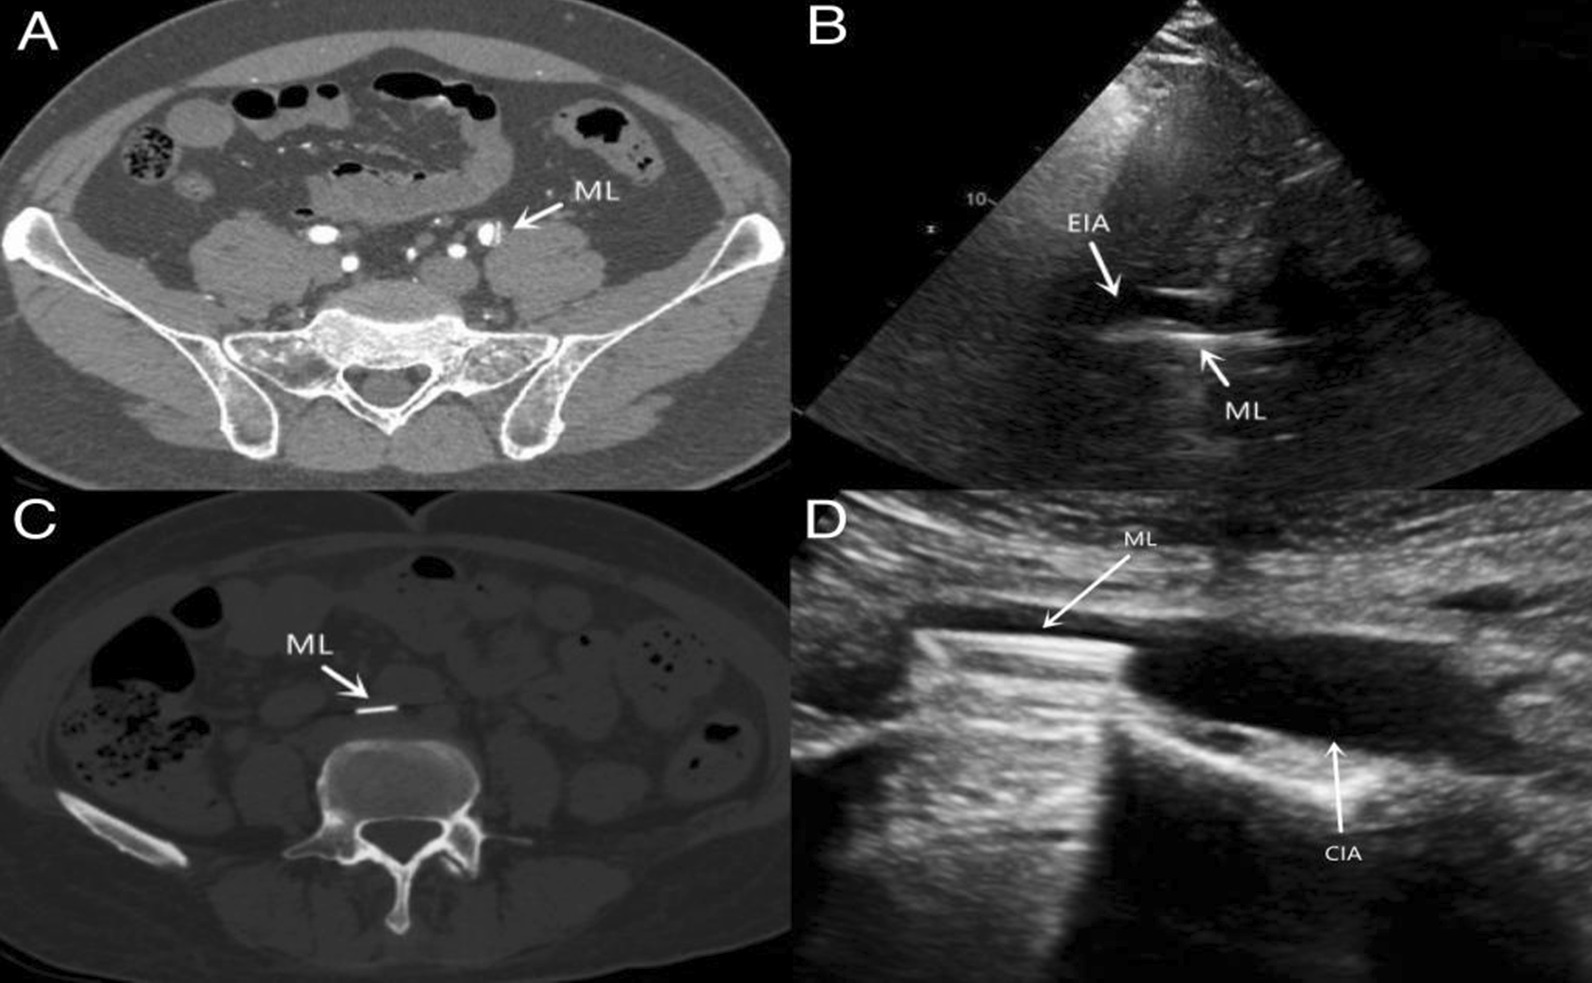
CT and ultrasound data of the escape bileaflet. A Ten years after the operation, CT showed that the detached aortic valve leaflet was located in the left external iliac artery, where the blood flow was unobstructed, and there was no obvious stenosis or peripheral hematomas. B Ten years after the operation, ultrasound showed that the detached aortic valve leaflet was located in the left external iliac artery, without thrombosis, and there was smooth blood flow; C Three months after the operation, CT showed that the detached mitral valve leaflet was located in the right common iliac artery, the leaflet was intact, and no peripheral hematomas were found. D Three months after surgery, ultrasound showed that the detached mitral valve leaflet was located in the right common iliac artery, the leaflet was intact, and no thrombosis or peripheral hematoma was found. ML: mechanical flap; EIA: External iliac artery; CIA: Common iliac artery; CT: Computerized tomography
